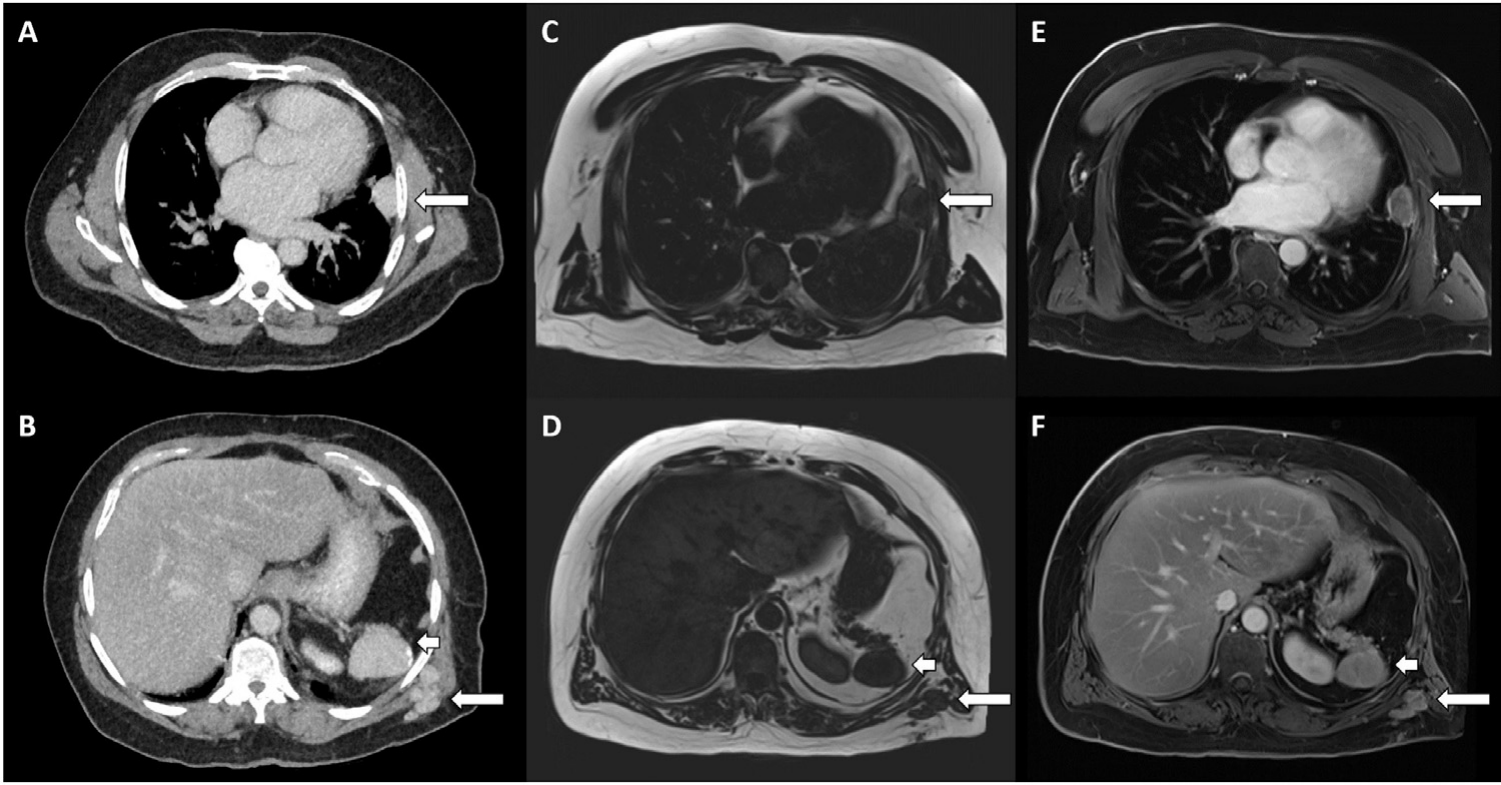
A 56-year-old man was admitted to the hospital for management of bilateral pulmonary emboli and investigation of dilated cardiomyopathy. His past medical history revealed a history of gunshot injury to the left chest requiring emergency laparotomy 30 years ago. IV-contrast enhanced CT imaging demonstrated homogeneously enhancing lobulated lesions at the left-sided pleura (A, long arrow) and left posterolateral chest wall (B, long arrow) with irregular partially-calcified spleen (B, short arrow). On Gadolinium-enhanced MRI imaging, the pleura and chest wall lesions demonstrated similar signal intensity and enhancement pattern to that of the spleen (D and F, short arrows), with central low signal intensity and hypointense rim on T1-weighted imaging (C and D, long arrows), and homogeneous enhancement on post-Gadolinium imaging (E and F, long arrows).
